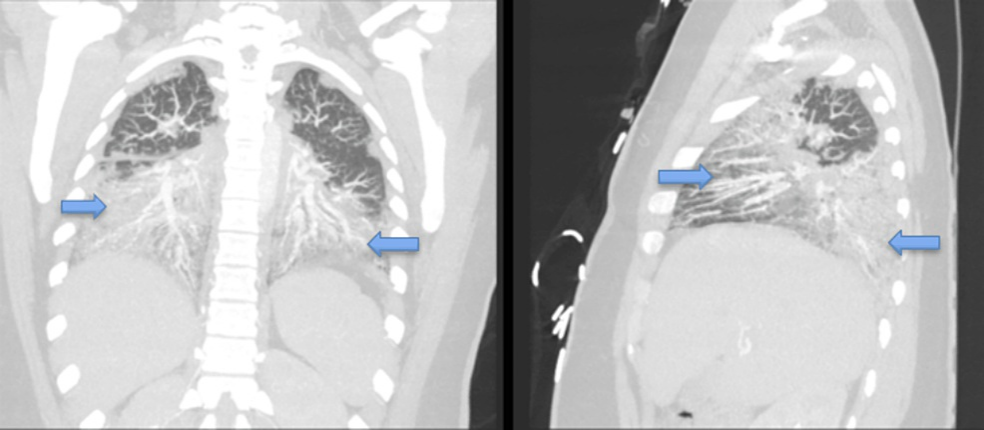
CT chest coronal and sagittal views CT chest coronal and sagittal views with bilateral airspace infiltration and opacities (blue arrows) related to infectious and inflammatory processes.
